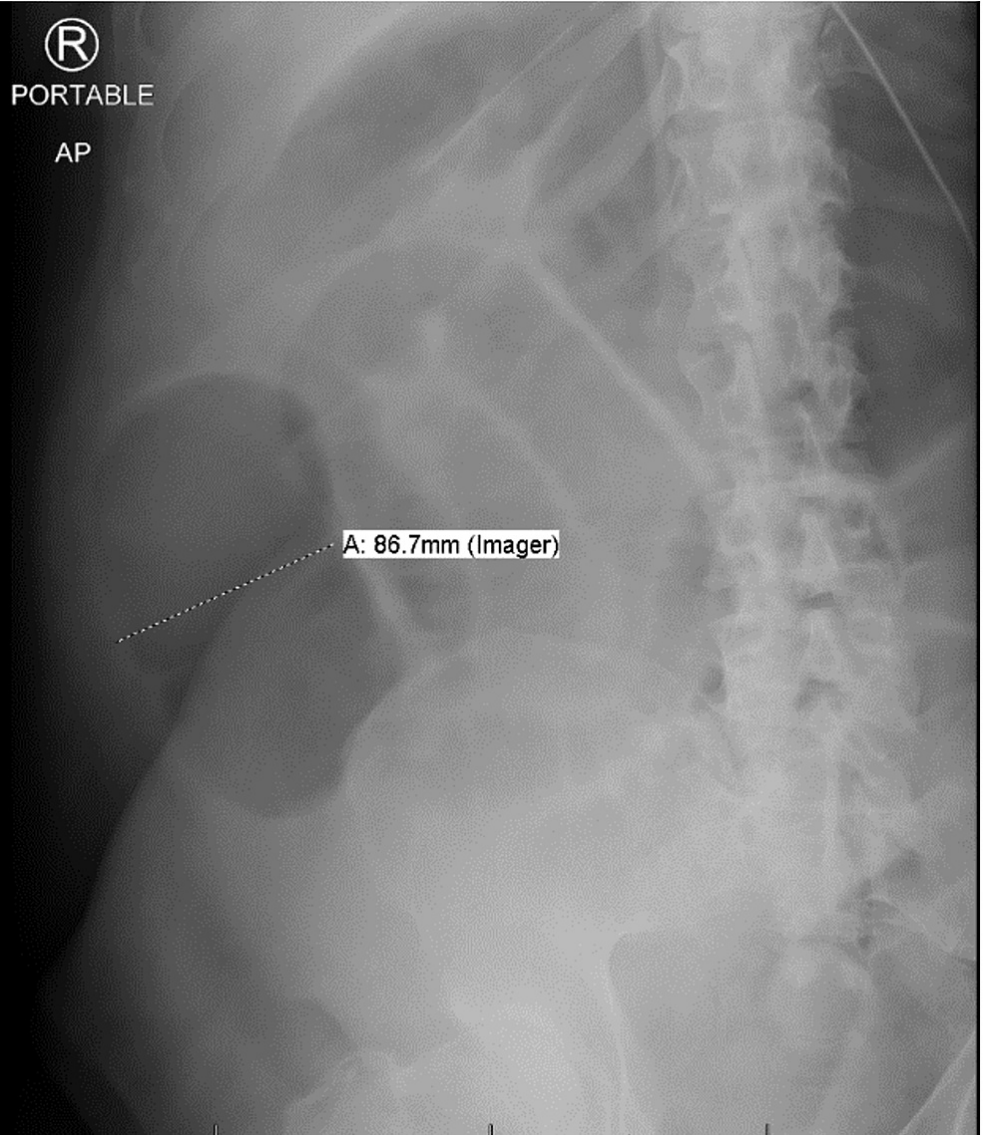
Portable abdominal x-ray demonstrating multiple dilated small bowel loops throughout the abdomen with the dilated cecum-ascending colon of more than 8 cm.
